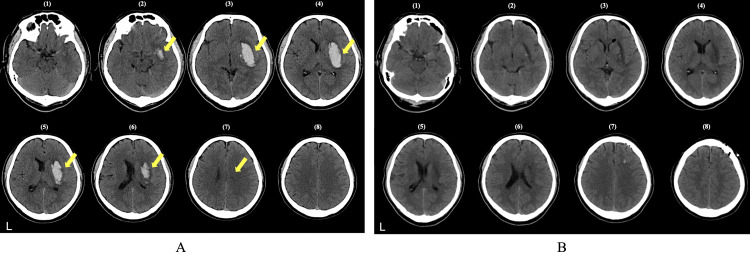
Preoperative and postoperative axial CT Images (A) Preoperative CT scans: Axial CT slices labeled (1) through (8), obtained upon admission, demonstrate a right putaminal hemorrhage (yellow arrows) measuring approximately 50 mL. The hematoma extends into the putamen, external and internal capsules, and the insular cortex. Each numbered slice corresponds to a successively higher axial level, highlighting the cranio-caudal extent of the hemorrhage. (B) Postoperative CT scans: Axial CT slices labeled (1) through (8), obtained 24 hours after emergency hematoma evacuation, show that the previously observed hyperdense hemorrhage is no longer visible (compare each slice with its corresponding slice in (A)), confirming successful evacuation of the hematoma. Mild postoperative changes, including subtle ventricular shifts, may be noted; however, there is no evidence of rebleeding or significant residual hematoma. Note: The slice numbers (1) through (8) in both (A) and (B) are matched by approximate axial levels to facilitate direct comparison of preoperative and postoperative scans. These CT images have been left-right reversed by MRIcron to maintain a consistent orientation with subsequent figures.
